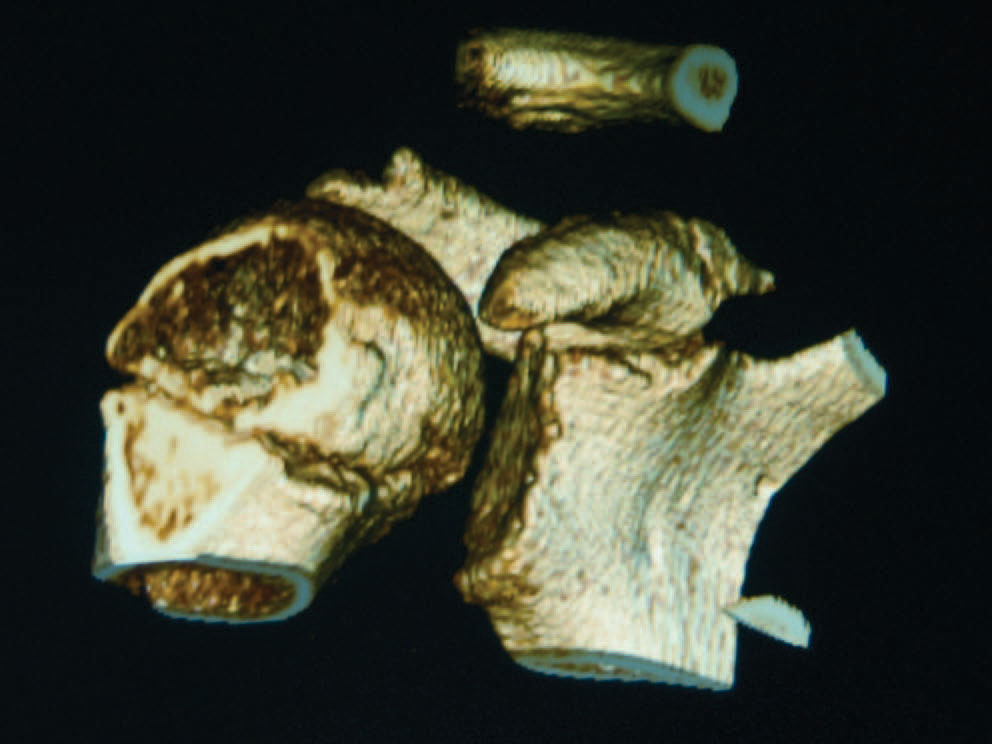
Three-dimensional reconstructions of the CT scan show a base of coracoid fracture with an intact epiphyseal plate.
